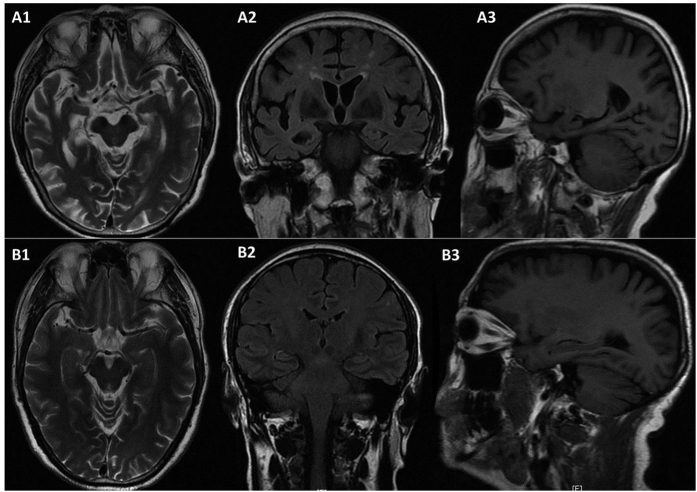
MRI scan of the patient and an age-matched control. (A1–A3) The cross sectional, coronal, and sagittal MRI of the patient revealed diffuse atrophy of the frontal lobe, temporal lobe and parietal lobe, especially the temporal lobe and the hippocampal. (B1–B3) The cross sectional, coronal, and sagittal MRI of the age-matched control appeared much normal.
